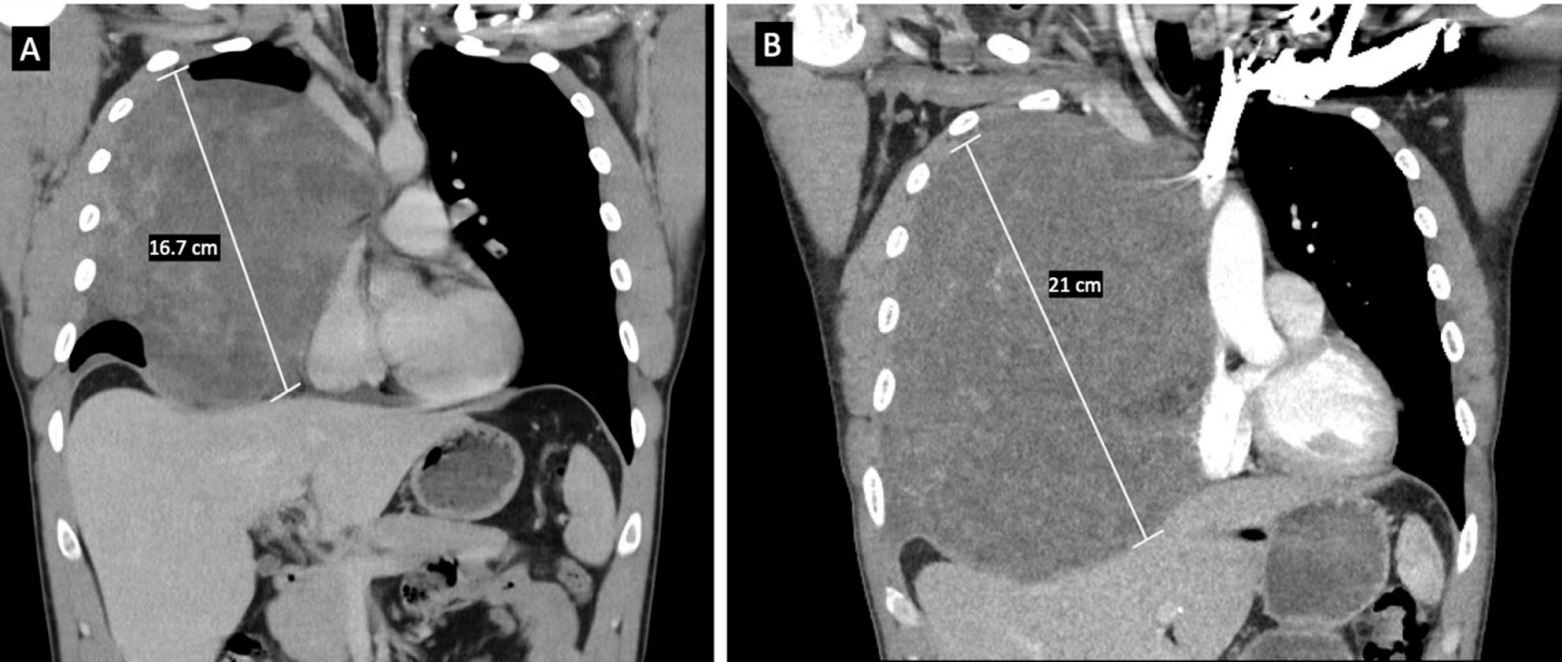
Chest computed tomography angiography (CTA) showing (A) initial 16.7 cm mass with smooth margins and compressed SVC. (B) Repeat chest CTA showing 21 cm mass after completing chemotherapy 2 months later
